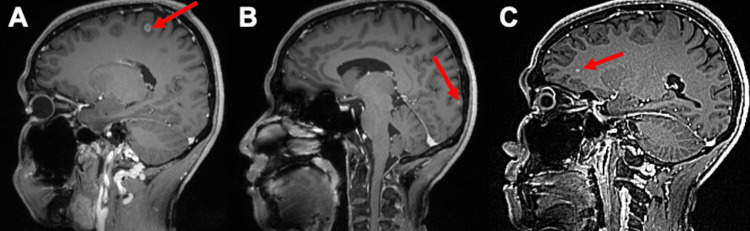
Brain MRI sagittal slices (A, B, C) Multiple small enhancing lesions within the cerebral hemisphere (red arrows).
